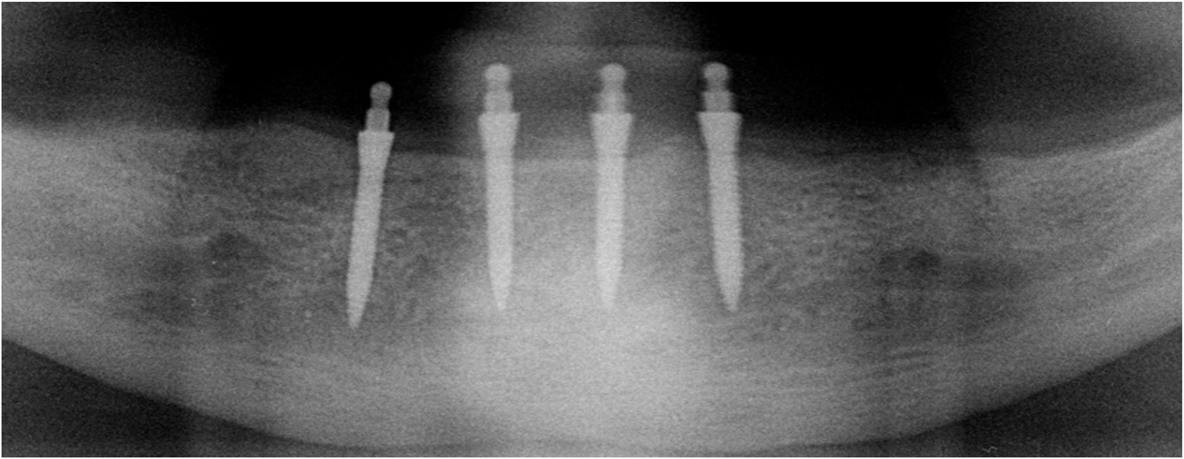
Excerpt from an exemplary preoperative radiography showing inserted MDIs
